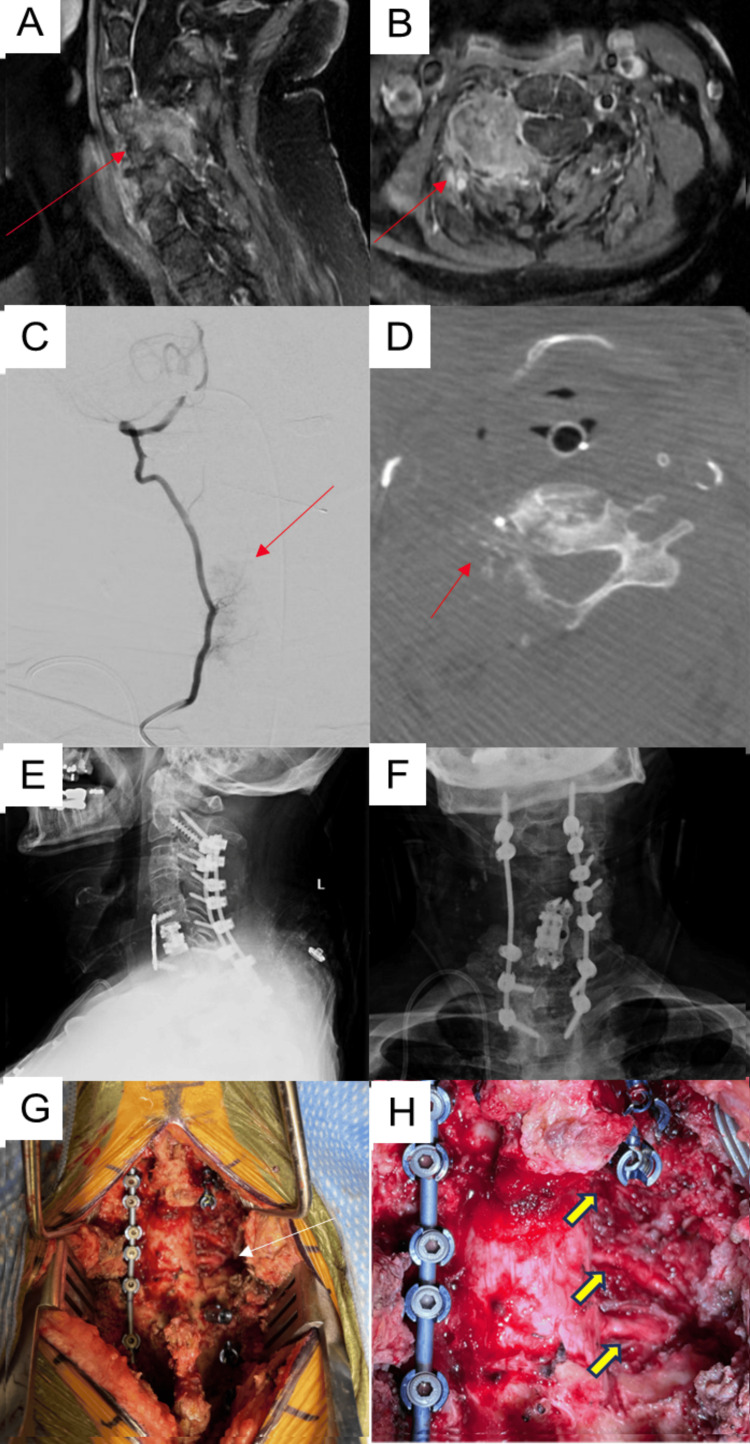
Tumor case A) Pre-operative sagittal T1-weighted post-contrast MRI of the cervical spine; B) Pre-operative axial T1-weighted post-contrast MRI of the cervical spine; C) Intra-operative sagittal angiogram demonstrating catheter placement within the proximal right vertebral artery; D) Intra-operative CTA using O-arm (Medtronic, Minneapolis, MN) demonstrating the complete envelopment of the right vertebral artery by tumor (red arrow); E) Post-operative lateral X-ray; F) Post-operative A/P X-ray; G) Intra-operative view at low magnification following tumor resection; H) Intra-operative view higher magnification following tumor resection demonstrating complete tumor resection and skeletonized thecal sac and nerve roots on the right side (yellow arrows).
